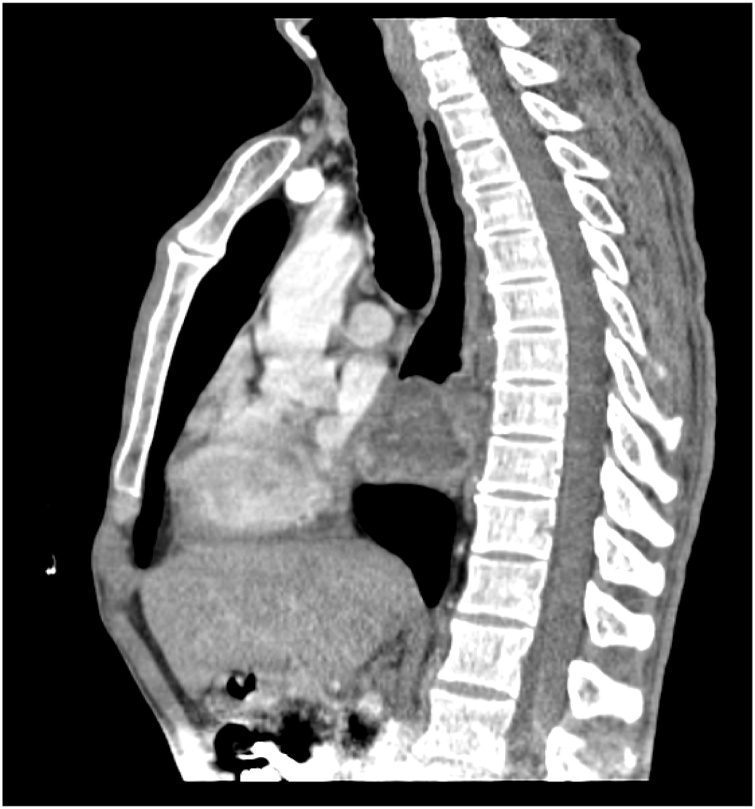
Sagittal view of computed tomography of the chest with contrast showing a low oesophagus well-circumscribed mass.
